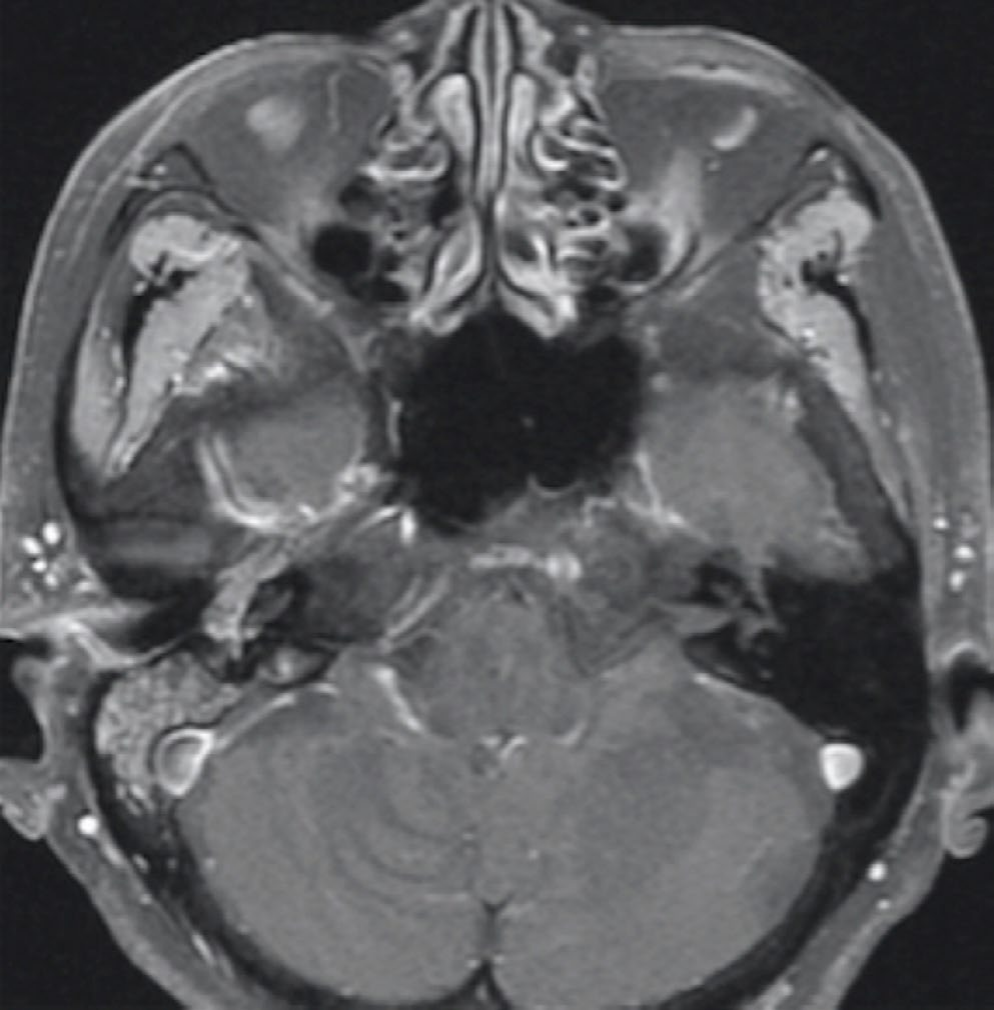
T1‐weighted axial MRI showed the tumor developed from the middle ear cavity to the external auditory canal
